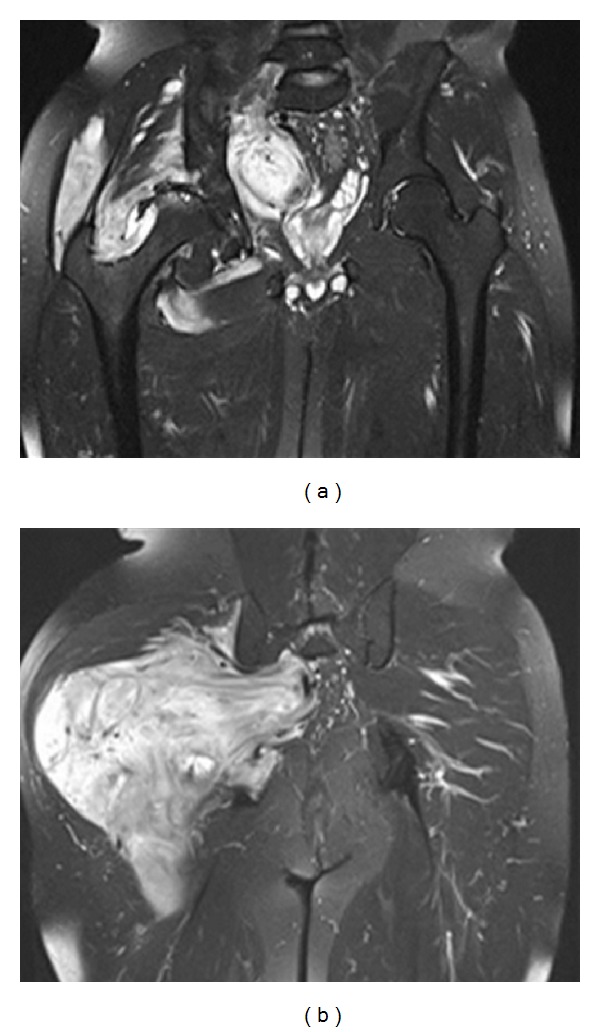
(a) The coronal T2-weighted demonstrates the vascular enhancing mass directly abuts the synovium of the right hip joint and wraps around the femoral neck. (b) The coronal T2-weighted demonstrates a large massive vascular enhancing mass which largely is posterior to the right hip.
